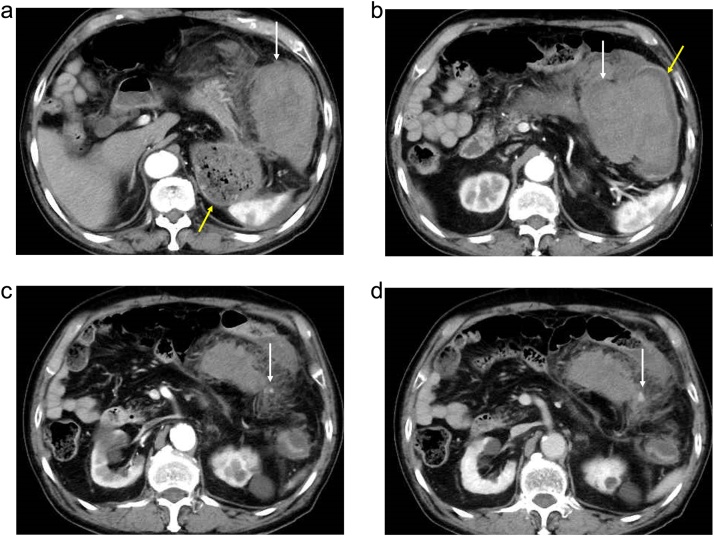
Abdominal contrast-enhanced computed tomography (CT) findings. (a) A mass (white arrow) with coexisting low- and high-density areas with a maximum diameter of 120 mm adjacent to the stomach (yellow arrow). (b) A mass (white arrow) adjacent to the transverse colon (yellow arrow). (c) Extravasation (white arrow) in the mass was observed in the arterial phase. (d) Extravasation (white arrow) spread in the portal phase.
